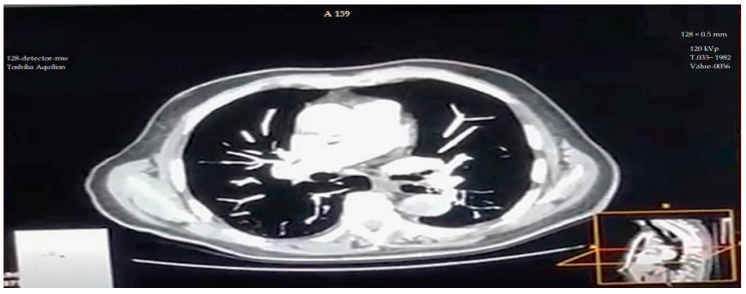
Representative lung-window CTPA image from the study cohort showing peripheral vascular enhancement. Axial lung-window CT pulmonary angiography slice at the basal level showing uniform contrast opacification of the segmental and subsegmental pulmonary arteries, with no evidence of acute embolism or motion artifacts. CT parameters: 120 kVp; 1.33 mm slice thickness; FC13 reconstruction kernel; WL: −600, WW: 1500; scale bar: 5 cm.
